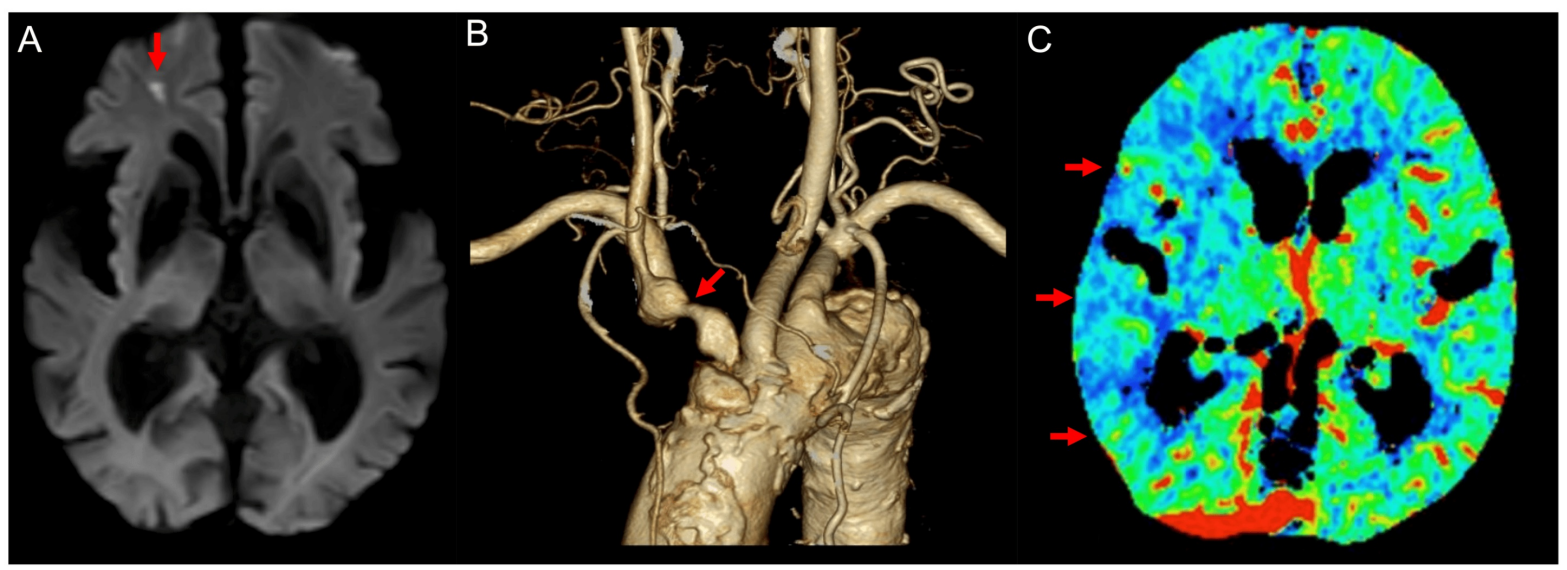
Preoperative images Magnetic resonance imaging on admission showed an infarct in the right frontal lobe (A) (arrow). Computed tomography angiography and perfusion imaging showed severe brachiocephalic artery stenosis (B) (arrow) and decreased cerebral blood flow (C) (arrows).
